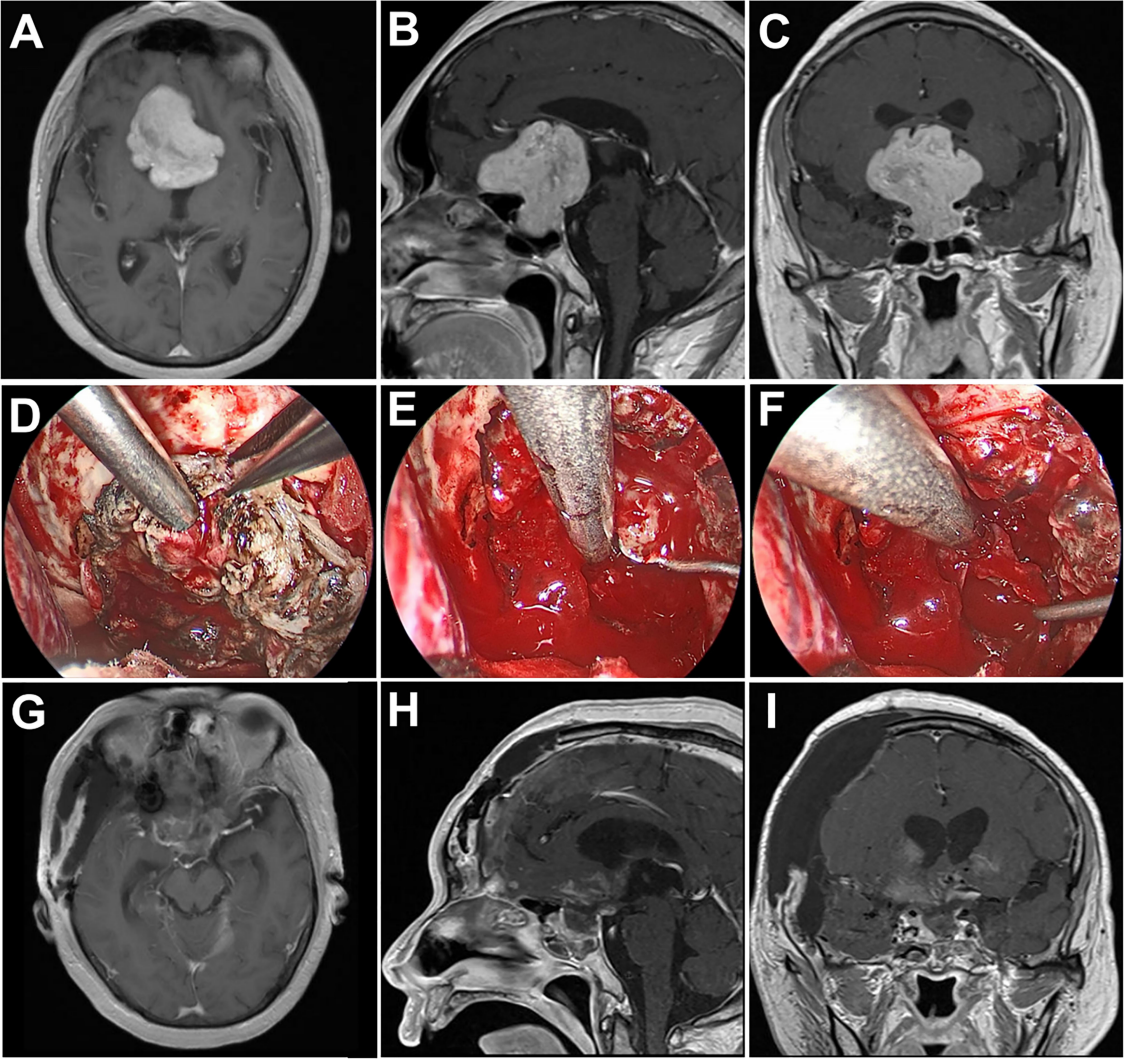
Case 4. Pre- and postoperative imaging findings and intraoperative images. (A–C) Preoperative axial (A), sagittal (B) and coronal (C) gadolinium-enhanced T1-weighted MRI images showing a giant pituitary adenoma with extensive suprasellar extension. (D) Intraoperative photograph showing that the diaphragma sellae is cut with micro-scissors. (E, F) Intraoperative endoscopic photographs showing the final view of the medial walls of both sides of cavernous sinus as well as the anterior wall of sella turcica after removal of the tumor. (G–I) Postoperative axial (G), sagittal (H) and coronal (I) gadolinium-enhanced T1-weighted MRI images showing gross total resection of the pituitary tumor.
